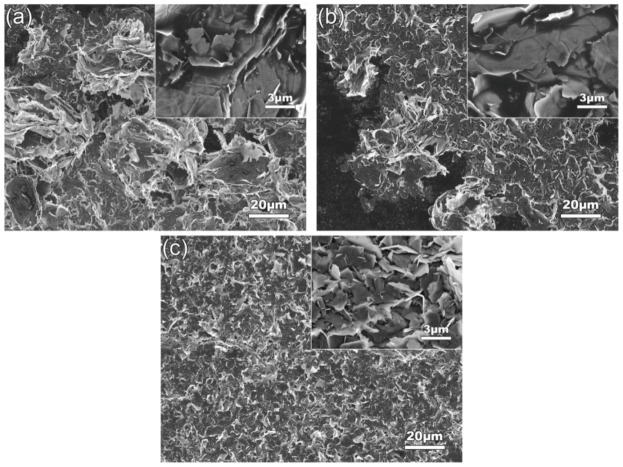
SEM images of GNPs ultrasonically dispersed at (a) 720 W, (b) 840 W, (c) 1080 W. Inserts are the corresponding high-magnification images.
